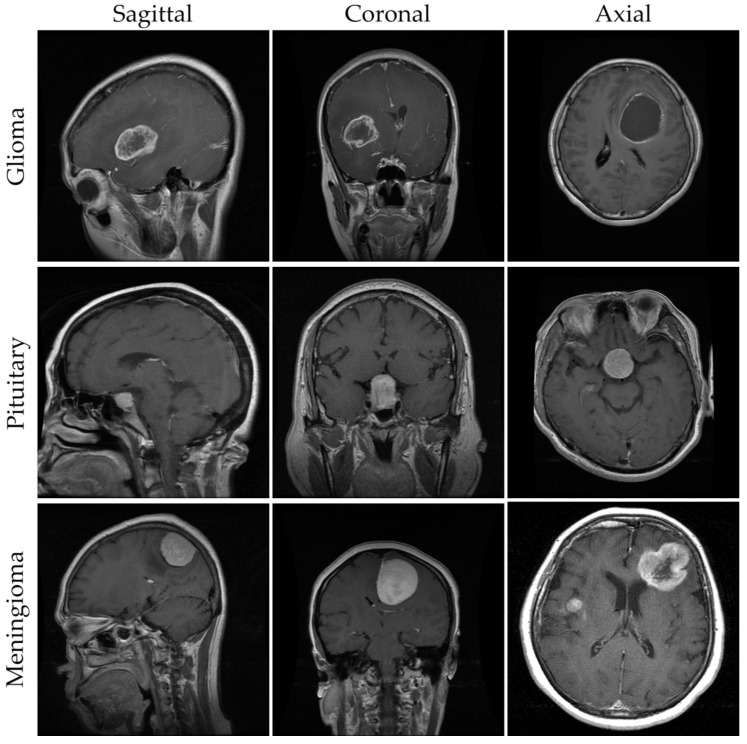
A sample of MRI images from the brain tumor dataset.
